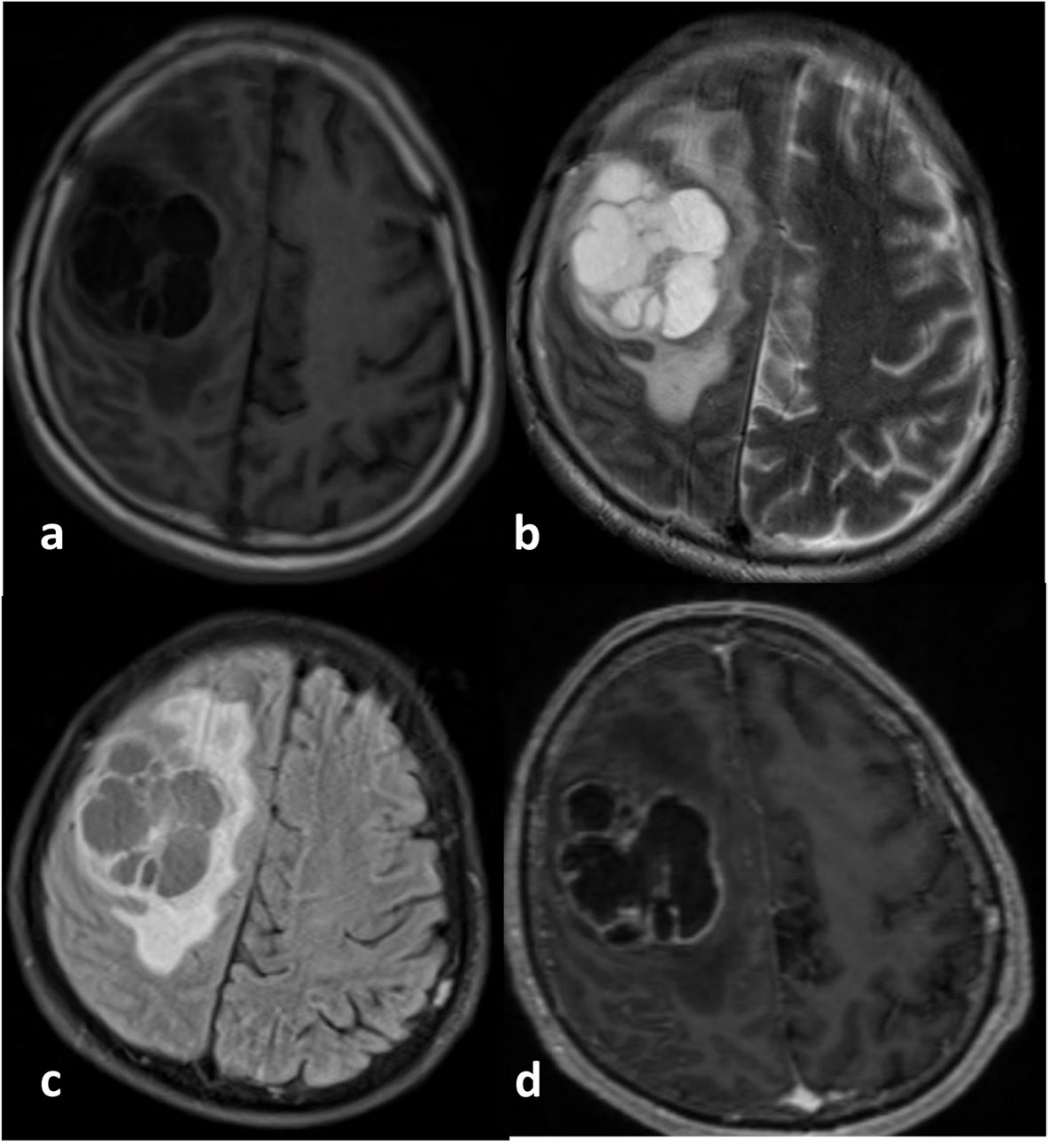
a Axial T1-weighted, (b) T2-weighted, (c) FLAIR, and (d) post-contrast administration T1-weighted MR images from a 60-year-old male with a Glioblastoma WHO CNS Grade 4, IDH-wildtype. There is a distinct and relatively thick area of enhancement on the edge of the necrotic area, with a cumulative size of about a third of the total tumor area. The abnormal FLAIR area appears slightly larger than the pathological intensity on the T1-weighted image. This glioma carries the IDH-wildtype marker. The tumor almost has no non-enhancing areas as it primarily consists of necrotic areas with a solid enhancing area at its edge. The longest diameter of the tumor size is relatively not too large, and the tumor's edema area seems limited to one hemisphere. This tumor is also MGMT-unmethylated
